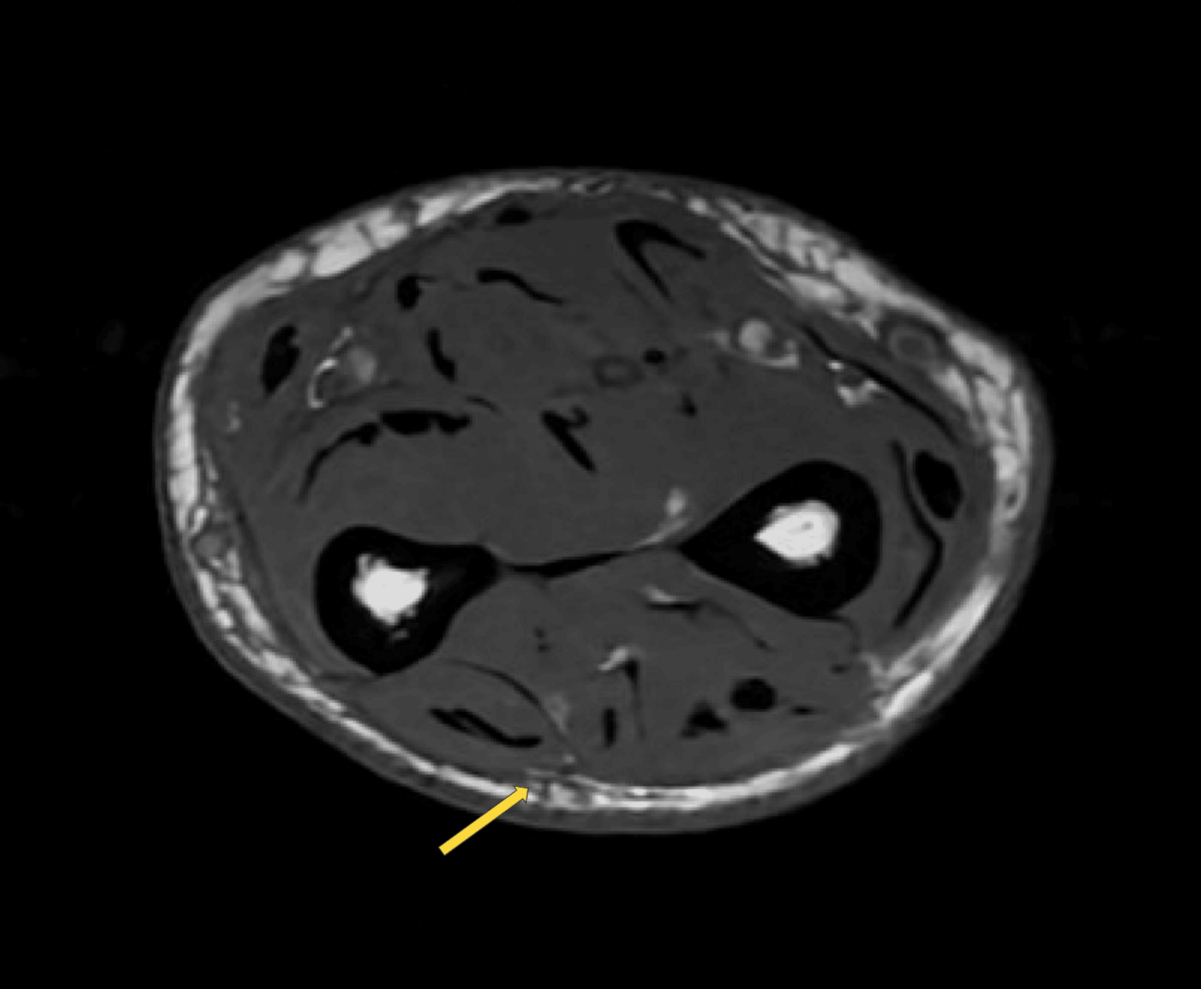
Nonspecific diffuse fasciitis Fascial thickening, increased signal intensity on fluid-sensitive sequence and enhancement after contrast administration on MRI.
